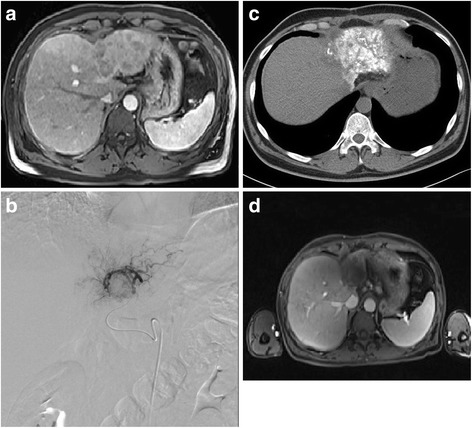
Partial response achieved after six sessions of TACE in a 66-year-old patient with HCC using mitomycin, lipiodol and DSM. a. Pre-treatment transverse contrast-enhanced T1-weighted MR image shows an intrahepatic liver lesion affecting both liver lobes. b. Prior to TACE procedure, angiographic image shows the presence of hypervascularity of liver tumor. c. CT after 3. TACE. Documentation of Lipiodol-deposition in the region of the HCC. d. Post-treatment transverse contrast-enhanced T1-weighted MR image after 6 sessions of TACE shows partial response of the intrahepatic lesion
